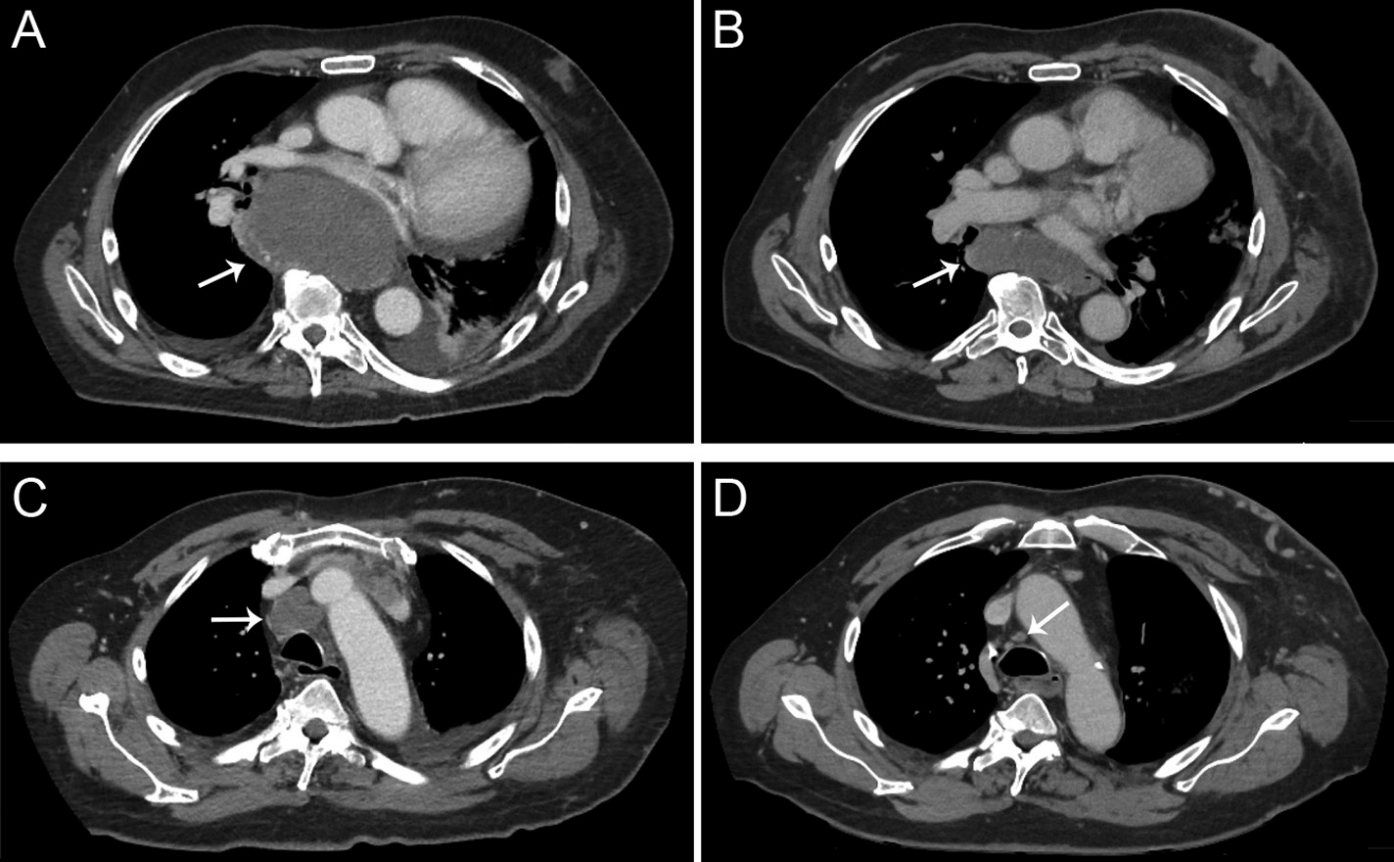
CT scan impressions of the (ongoing) partial response (according to RECIST) on 7 months of lorlatinib treatment. The sum of four target lesions has decreased from 192 mm at baseline to 75 mm; two of these target lesions are shown in the figure. A subcarinal lymph node metastasis reduced from 64 mm at baseline (A) to 28 mm after 7 months lorlatinib (B) in diameter. A pretracheal lymph node metastasis showed a reduced diameter from 29 mm at baseline (C) to 8 mm after 7 months lorlatinib treatment (D).
